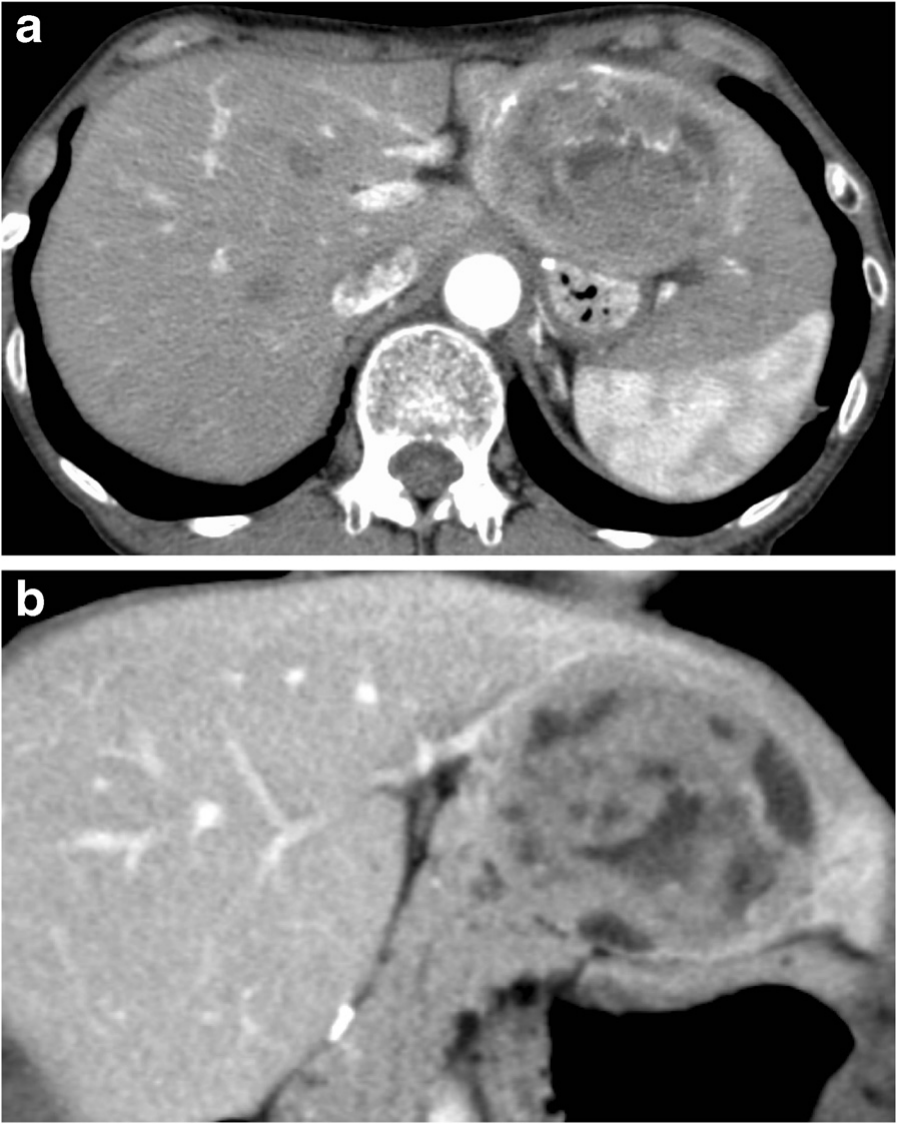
CT scan showing a large and round mass in the left hepatic lobe. The irregular low-density area resembling a mosaic pattern was found during the arterial (a) and portal phases (b)
